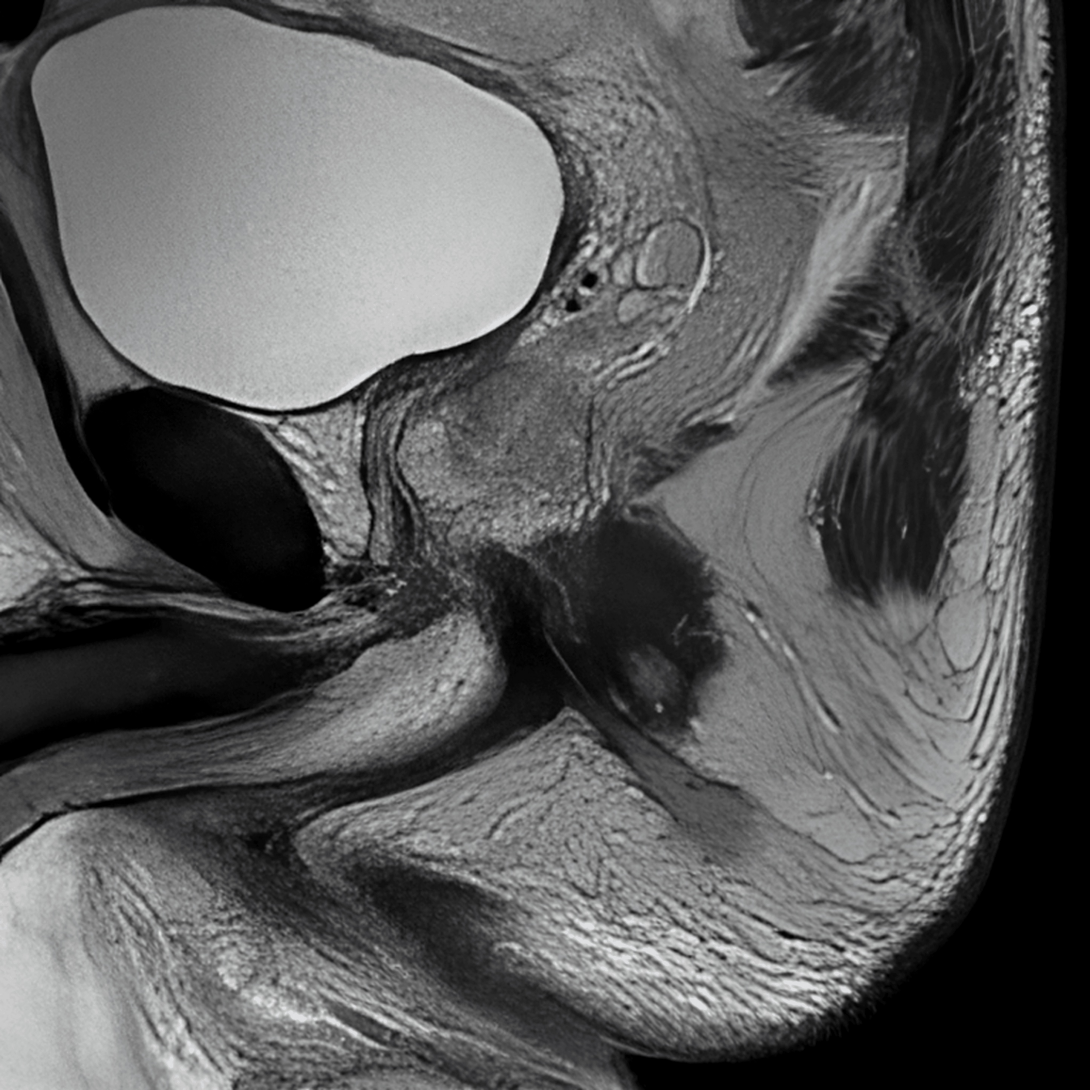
Sagittal T2-weighted MRI of the pelvis demonstrating a hyperintense tract consistent with a fistulous pathway
